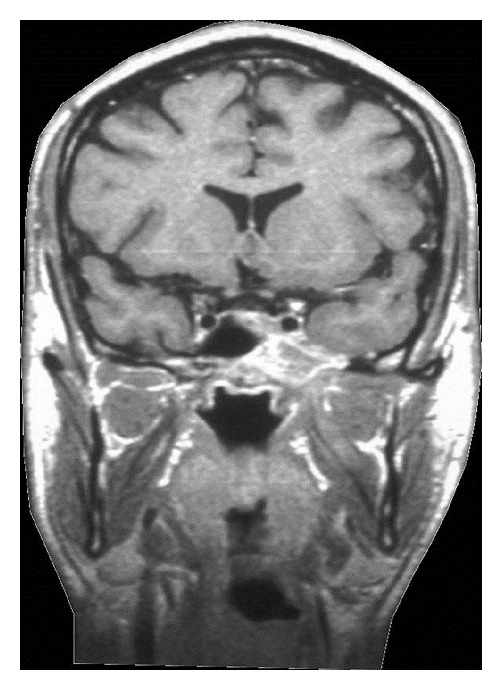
Cranial MRI 5 weeks after radiotherapy.
